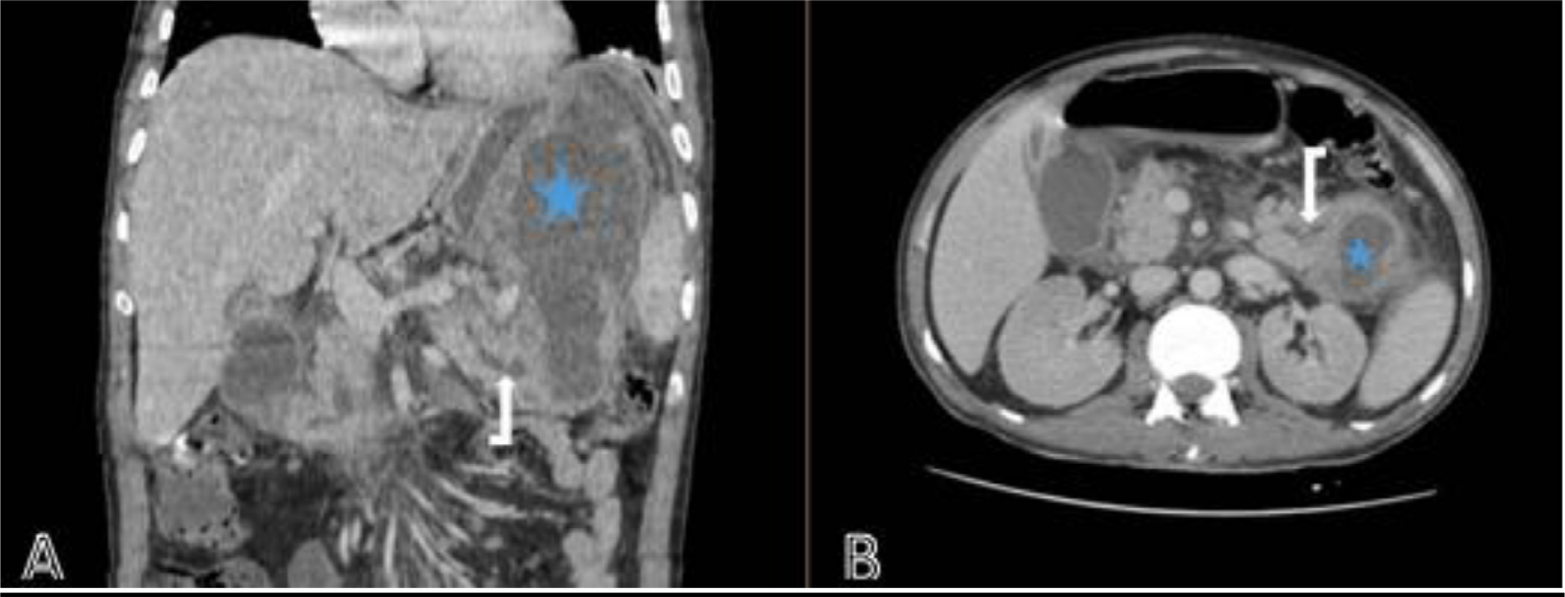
Coronal section (B) axial section of a contrast enhanced abdominal CT scan revealing signs of stage E pancreatitis associated to a cystic mass (blue star) extending between the pancreatic tail and the gastric region measuring approximately 15 × 6 cm. This mass seems to communicate with the Wirsung pancreatic duct which is dilated (white arrow).
